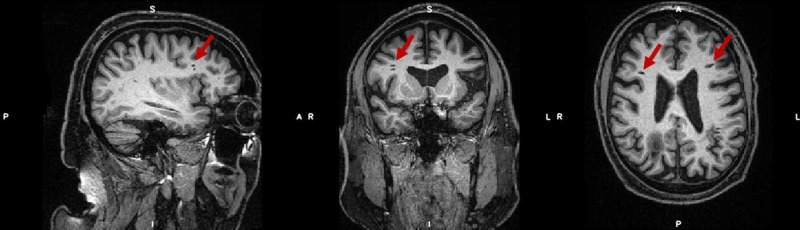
MRI scan (T1-MPRAGE) performed at 3T during research visit Position shows the smaller focal lesions in the frontal lobe as indicated by the red arrows. MRI: magnetic resonance imaging; T1‐MPRAGE: T1‐weighted magnetization‐prepared rapid gradient echo.
